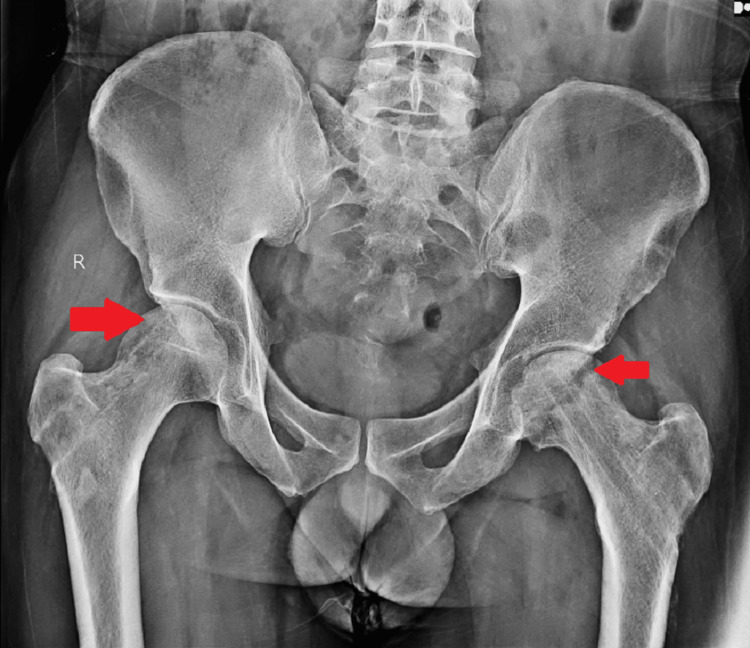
Two-month post-operative AP radiograph of the pelvis Core decompression with reverse bone grafting done and osteoinduction seen on both sides where grafts were inserted.
